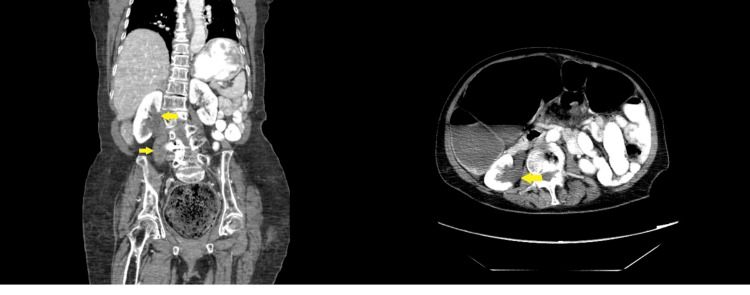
CT abdomen pelvis showing right hydronephrosis and hydroureter, likely resulting from external compression due to the significant colonic distension.
